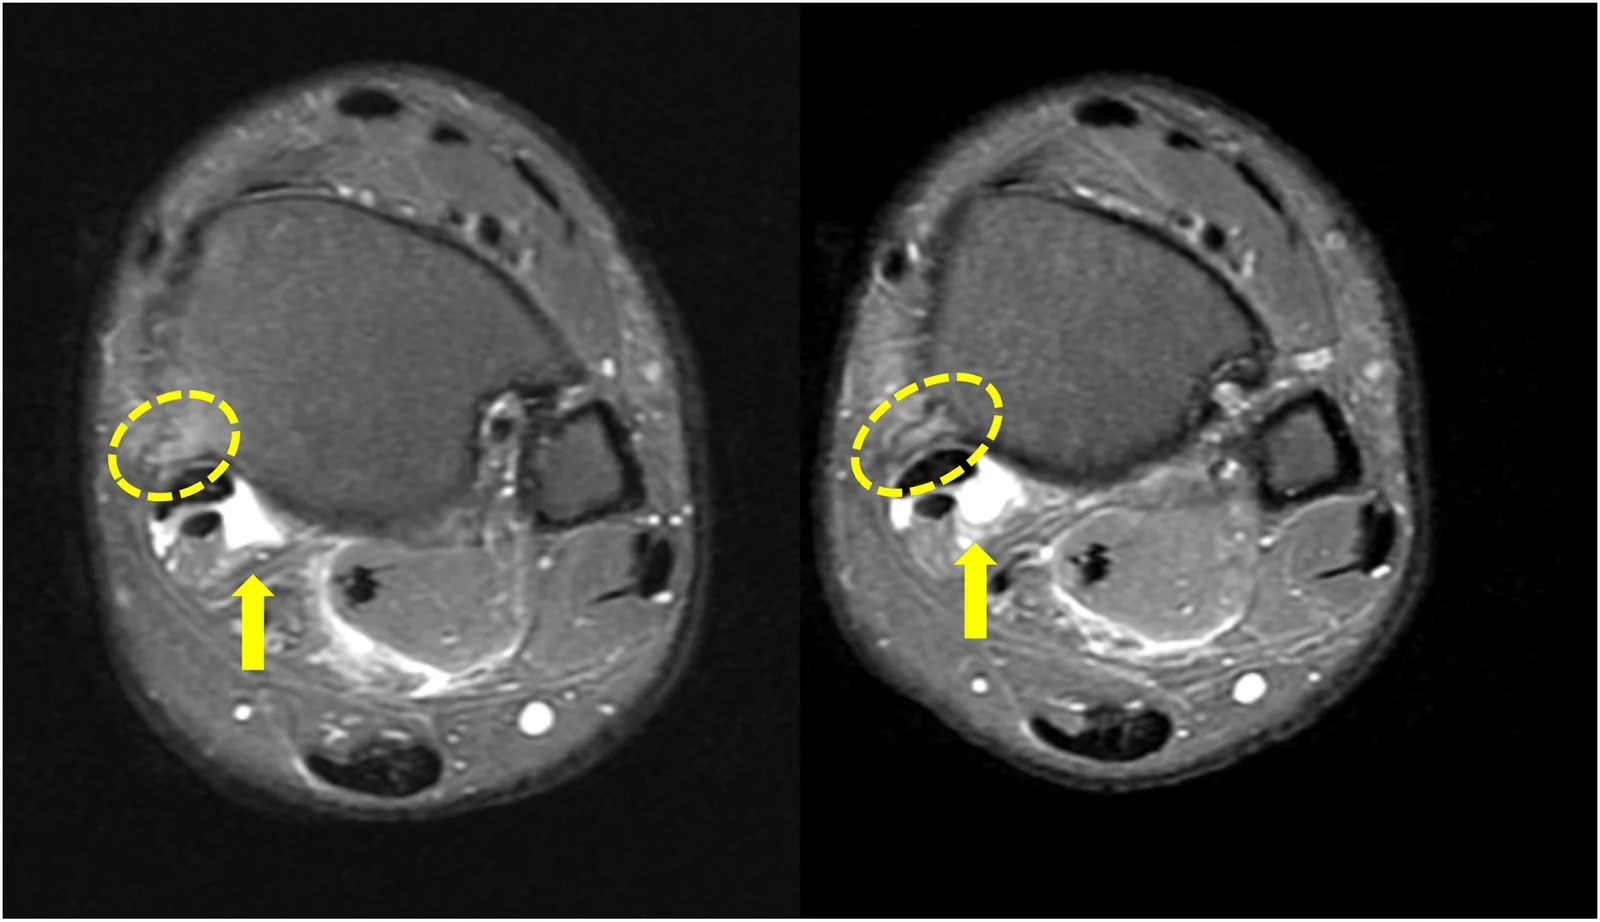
Preoperative MRI of the left ankle obtained approximately five months prior to presentation at our institution. The dashed circle indicates cortical thickening with osteophytic spurring at the posteromedial tibial margin; the yellow arrow identifies peritendinous fluid accumulation.
